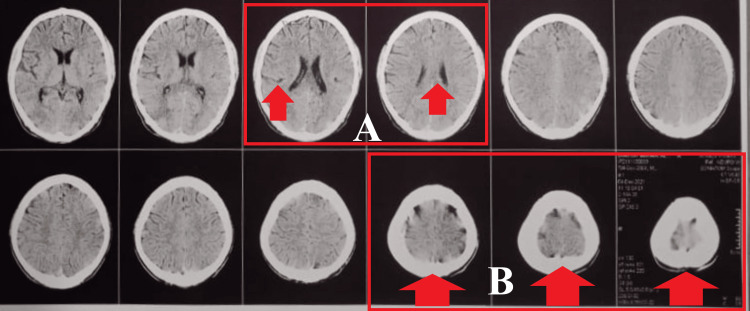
A: MRI scan that shows hyper-intense foci near the corpus callosum of the brain; B: MRI showing subdural hematoma. MRI: magnetic resonance imaging.
